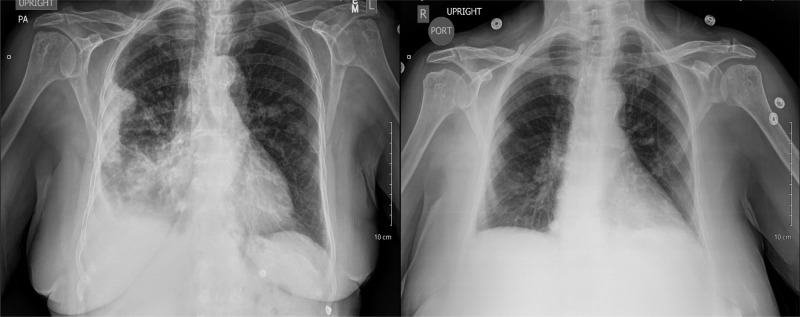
Posteroanterior and portable chest X-ray (Left figure) Posteroanterior chest X-ray showing dense peripheral right lung pleural-based opacity with blunting of the right costophrenic angle. Interstitial prominence of the right lung is present with right lower lung opacity. Nodular opacities are seen in the left midlung. (Right figure) Portable chest X-ray 2 months prior to the image at the left, showing no focal airspace opacity. A calcified nodule is seen at the right cardiophrenic junction which is unchanged compared to previous images.
